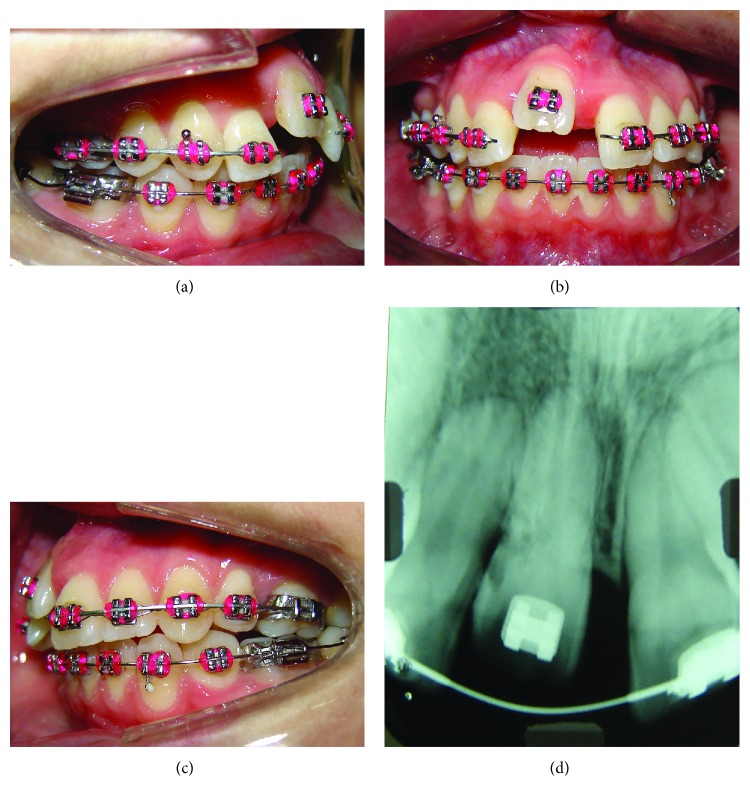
Intrabuccal photographs and periapical radiograph before surgery.
